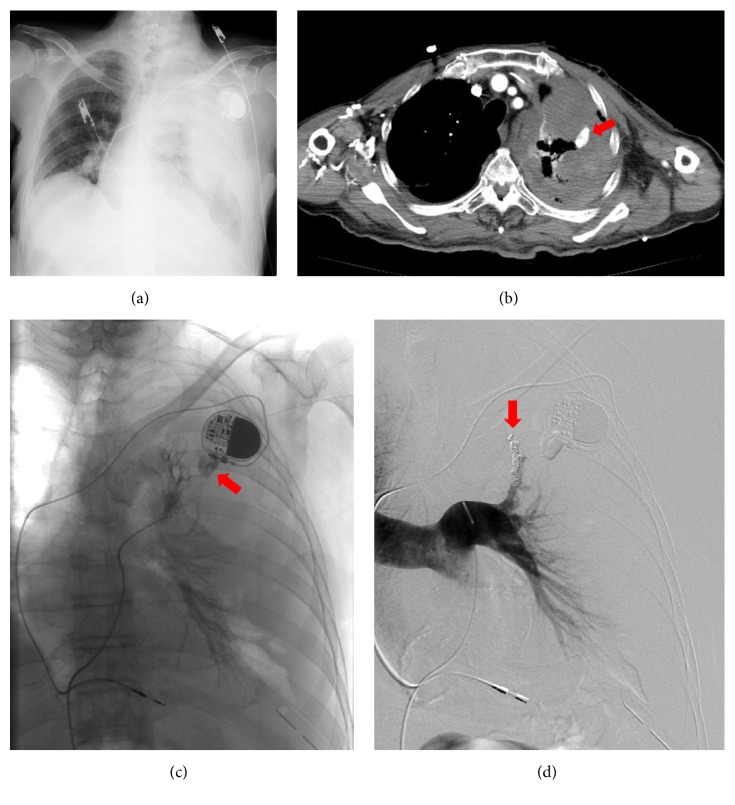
A 90-year-old man received a permanent implanted pacemaker. After the procedure, the patient's blood pressure steadily decreased. Left hemothorax and laceration of the pulmonary arterial branch of the left upper lobe were confirmed. (a) Chest radiograph showed a left hemothorax. (b) Contrast-enhanced computed tomography (CT) showed a confined cavity (pseudoaneurysm) of a branch of the upper lobe pulmonary artery (arrow). (c) Angiography demonstrated bleeding from the perforated pulmonary arterial branch of the left upper lobe (arrow). (d) Transcatheter arterial embolization (TAE) was performed using microcoils (arrow). Digital subtraction angiographic image taken after coil embolization discloses coils (arrows) in the upper lobe pulmonary artery and no further opacification of the pseudoaneurysm.
